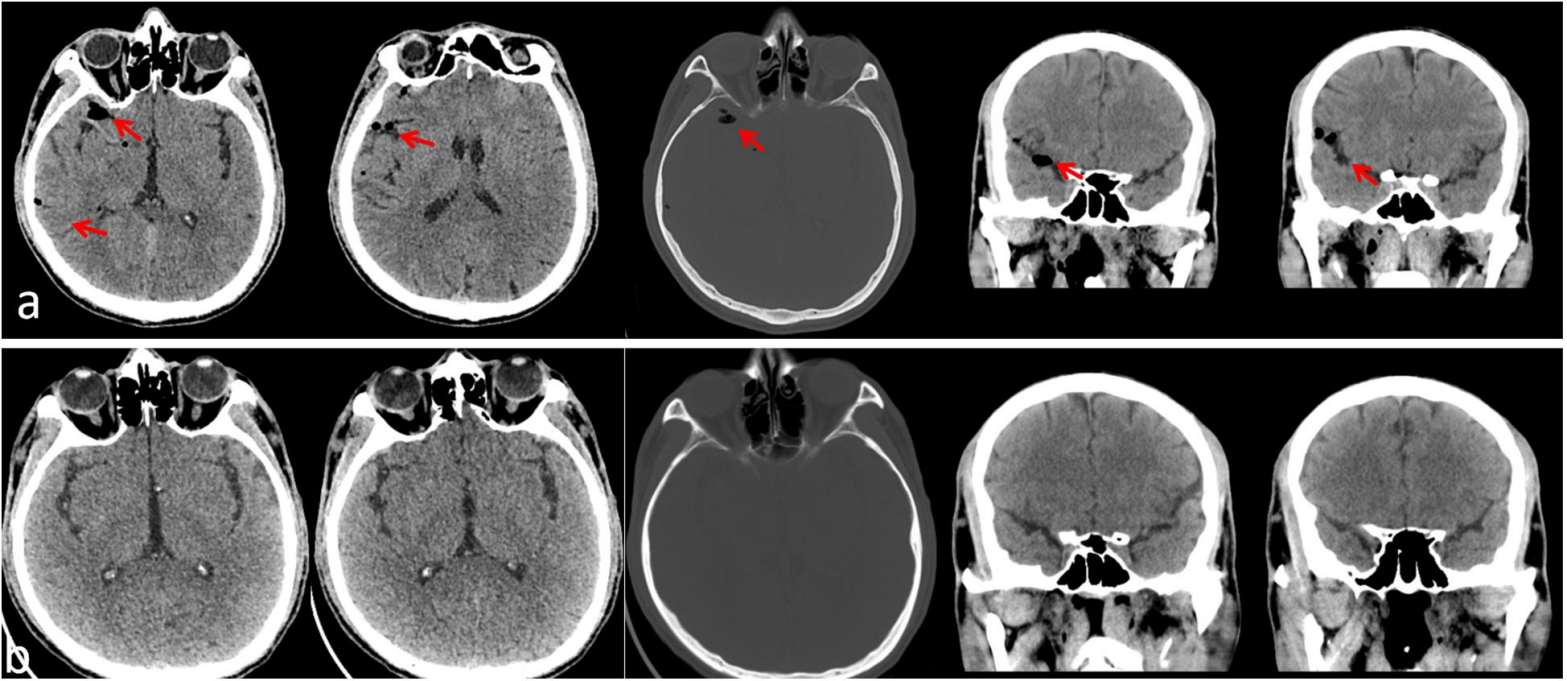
Postoperative head CT scan 2 h after surgery, showing gas in the right frontal brain sulcus, with normal ventricular system and cisterns, and no midline shift (a); postoperative head CT scan on the 5th day showing resolution of pneumocephalus (b).
